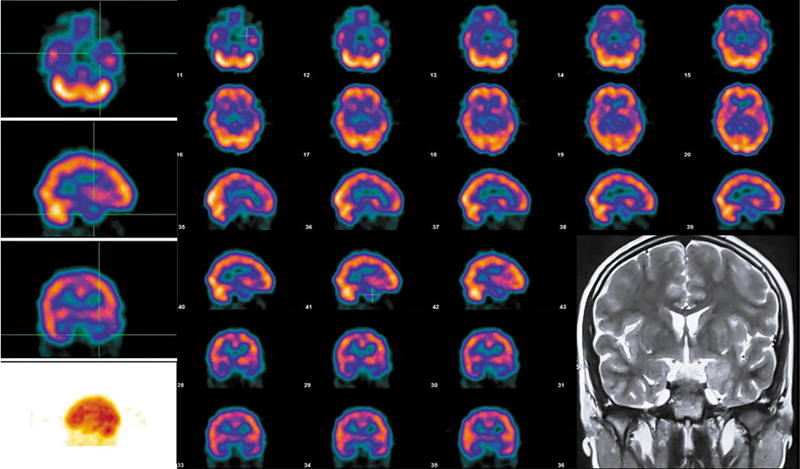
Interictal 99mTc-ethyl cysteinate dimer (ECD) brain perfusion single photon emission computed tomography (SPECT) in a 12-year-old male child with right-sided focal epilepsy of 8 years' duration, magnetic resonance imaging (MRI) coronal sections show left-sided mesial temporal sclerosis. SPECT images showing moderate to severe hypoperfusion involving left medial temporal cortex and mild hypoperfusion involving right medial temporal cortex.
